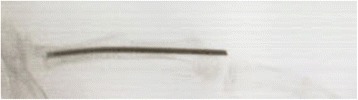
Lateral X-ray of the intramedullary compressed femur immediately postoperatively
